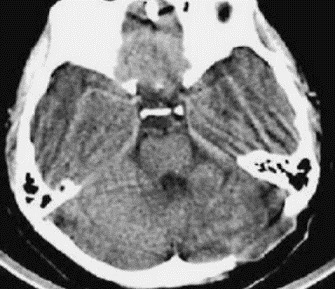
Brain CT with contrast 20 months after surgery and antibacillary treatment showing resolution of the lesion, the fourth ventricle is normally visible
